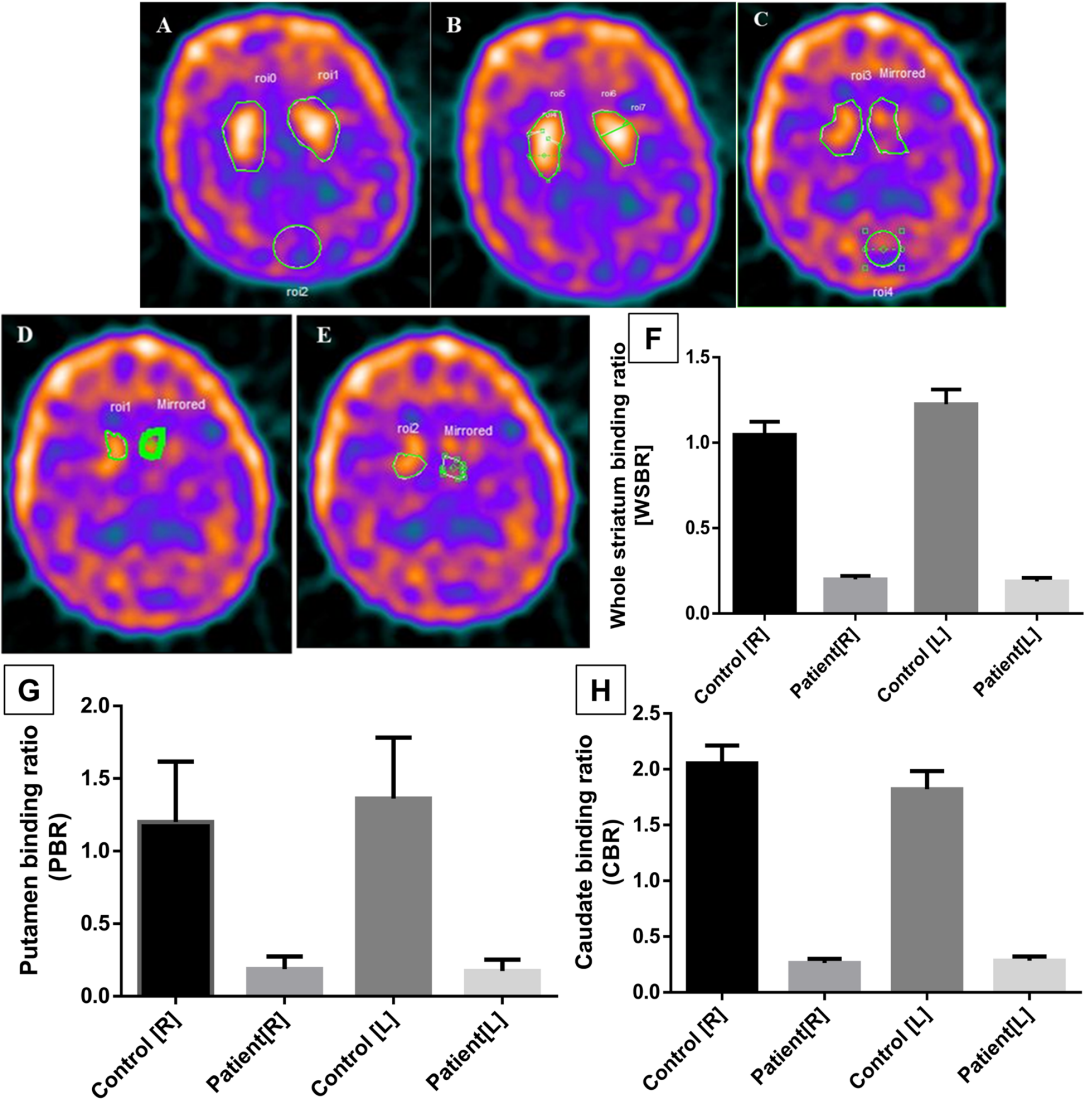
^99m^Tc TRODAT-SPECT imaging of healthy age-matched control and Parkinson’s disease patients. A, B Representative images for the basal ganglia of the healthy age-matched control with the typical striatal region of interests (ROIs): A whole striatum (upper marked areas) and occipital (lower circle), B left and right caudate (upper marked areas) and left and right putamen (lower marked areas). C, D, and E Representative image for the basal ganglia of Parkinson’s disease patients with a typical striatal region of interest (ROI): C whole striatum (upper marked areas) and occipital (lower circle), D left and right caudate, E left and right putamen. Comparison of the whole striatum binding ratio (F), putamen binding ratio (G), and caudate binding ratio (H) between PD and HC
